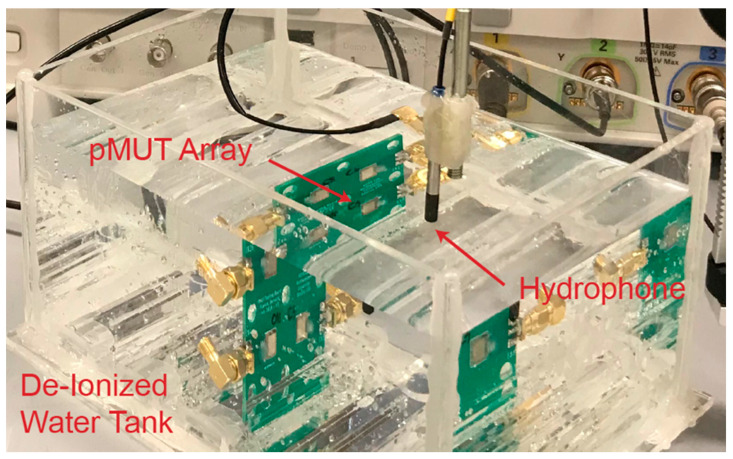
De-Ionized (DI) water tank for ultrasonic experiment measurements. The transmission is done between a pMUT array and an ultrasonic hydrophone (Teledyne TC4038) for reference. Given the small dimensions of the tank, there will be some interference due to the reflections at the interface, creating a multipath. Absorptions layers can be added to the wall to reduce the multipath phenomena.
